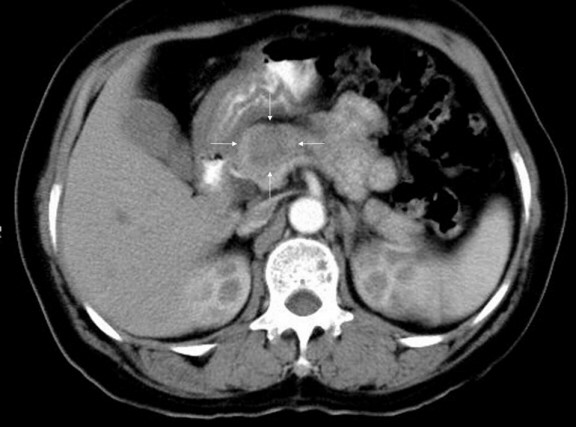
Contrast enhanced abdominal computed tomography showing an ill defined mass revealing faint inhomogeneous low density in the body of the pancreas.
